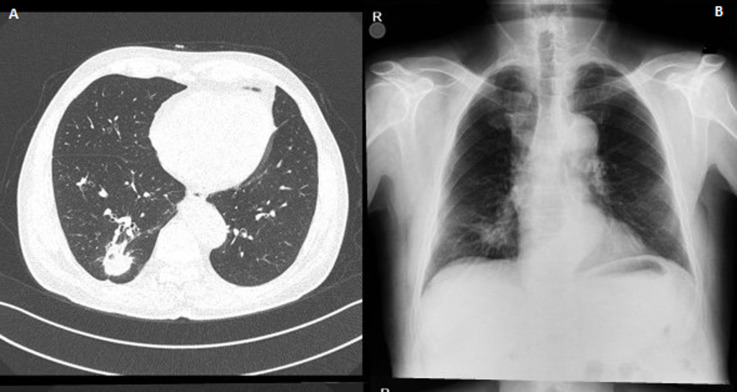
an irregular density was observed in the lower segment of the right lung on the chest X-ray (A), solid mass with speculated irregular contours mimicking malignancy, in the posterior basal segment of the lower lobe of the right lung (B)
